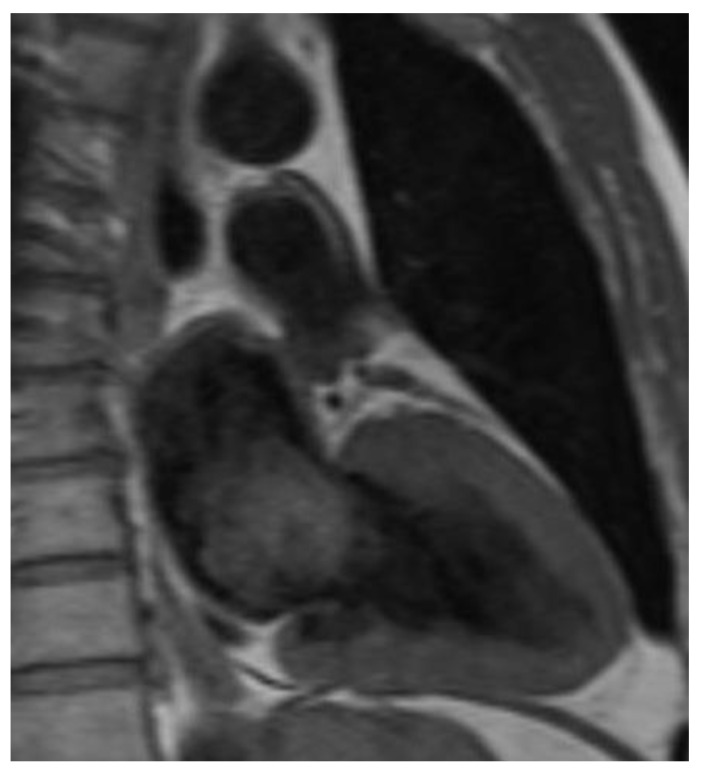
Magnetic resonance imaging of a left atrial CM.
